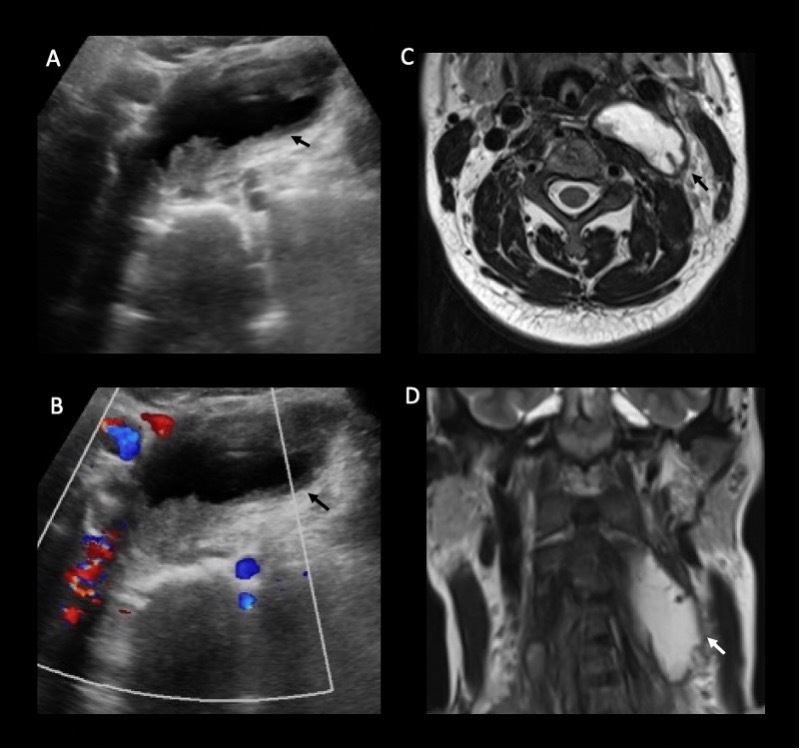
Images A and B are transversely oriented ultrasound images demonstrating a large left predominantly cystic retropharyngeal abscess measuring 8 × 4 x 2 cm (arrows). Images C and D, both *T_2_* weighted MRI sequences, demonstrate this retropharyngeal abscess within its left paravertebral location.
